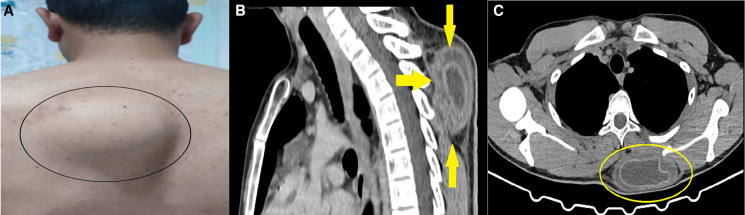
(A) Physical examination of a mass in the left scapular region (black circle). (B) Sagittal computed tomography (CT) image revealing a well-defined cystic lesion of the posterior thoracic wall (yellow arrows). (C) Axial CT image revealing the superior portion of the cystic mass (yellow circle), confirming its subcutaneous location measuring ∼9 cm. The mass is confined to the subcutaneous soft tissues without invasion of underlying muscles or bone.
